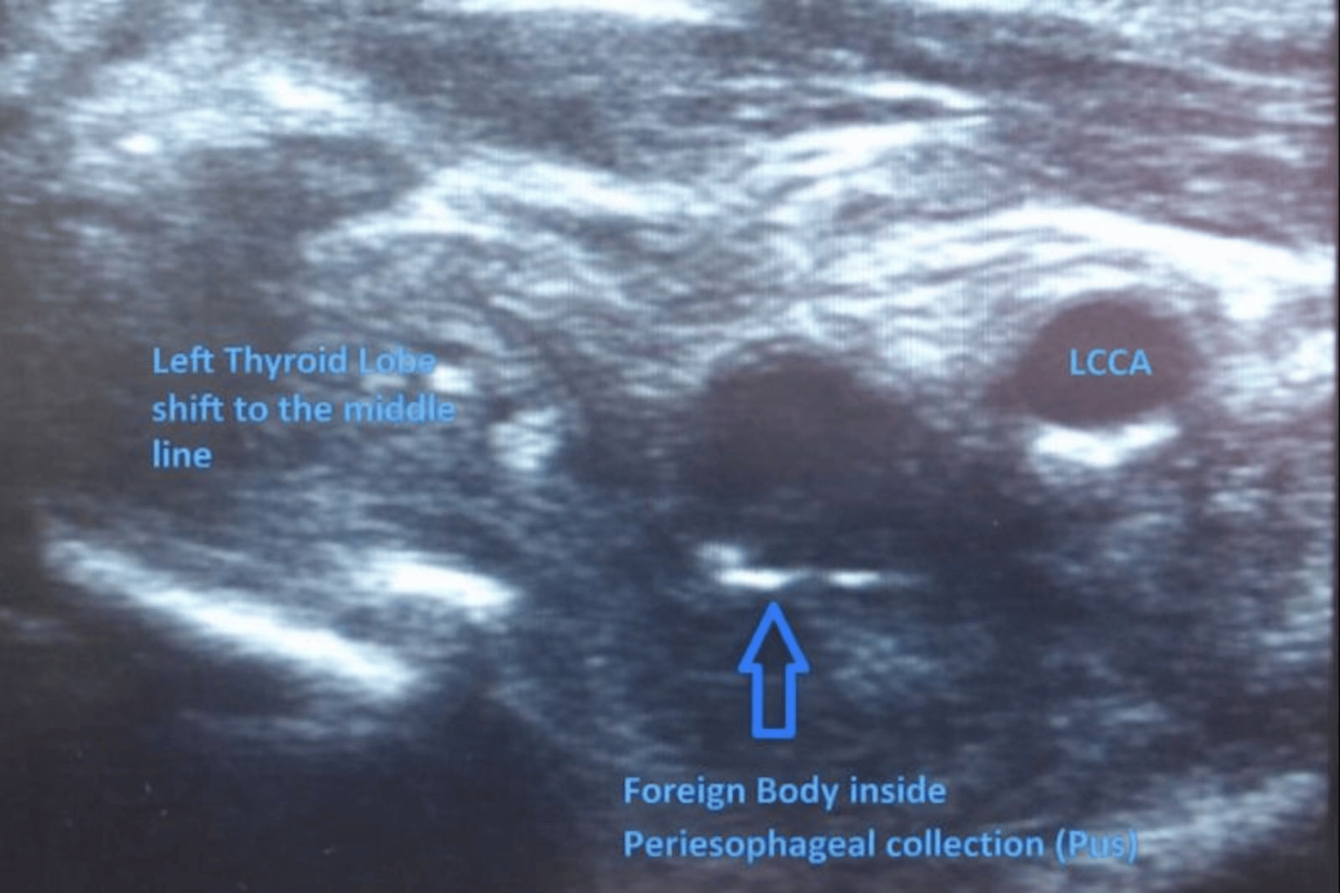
Point-of-care ultrasound of the left cervical region demonstrating a complex peri-esophageal fluid collection with internal air pockets and a linear hyperechoic structure consistent with a retained foreign body external to the esophageal wall (arrow) LCCA, left common carotid artery
